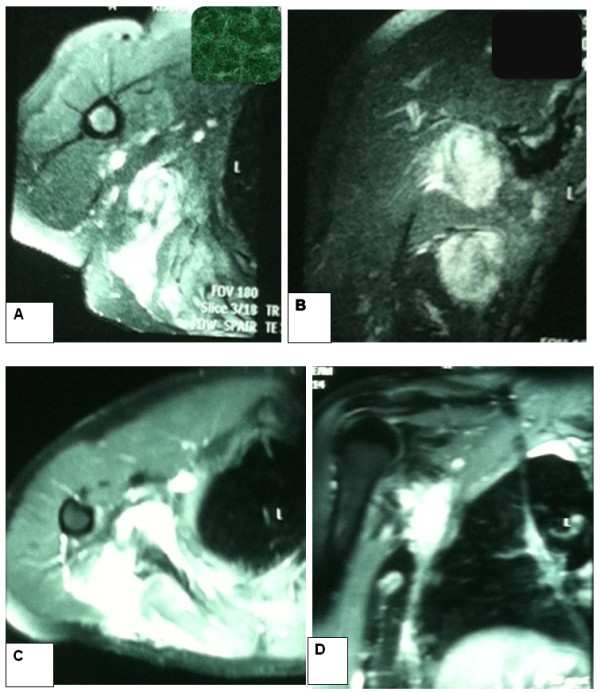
Pre and post second surgery magnetic resonance imaging. Axillary magnetic resonance imaging performed before second surgery (A-B) and after surgery (C-D). Magnetic resonance imaging axial and sagittal cut (A-B) showing soft-tissue mass recurrences measuring 126mm on high axis in right chest wall, hypointensity T1 and hyperintensity T2, contact with axillary pedicle and brachial vessels homolateral. These vessels remains permeable and are not involved; a second similar soft tissue mass of right chest wall in posterior areas measuring 61mm in diameter was noted. After surgery, right auxiliary magnetic resonance imaging (C-D) was performed and showed a residual tumor measuring 21mm.
